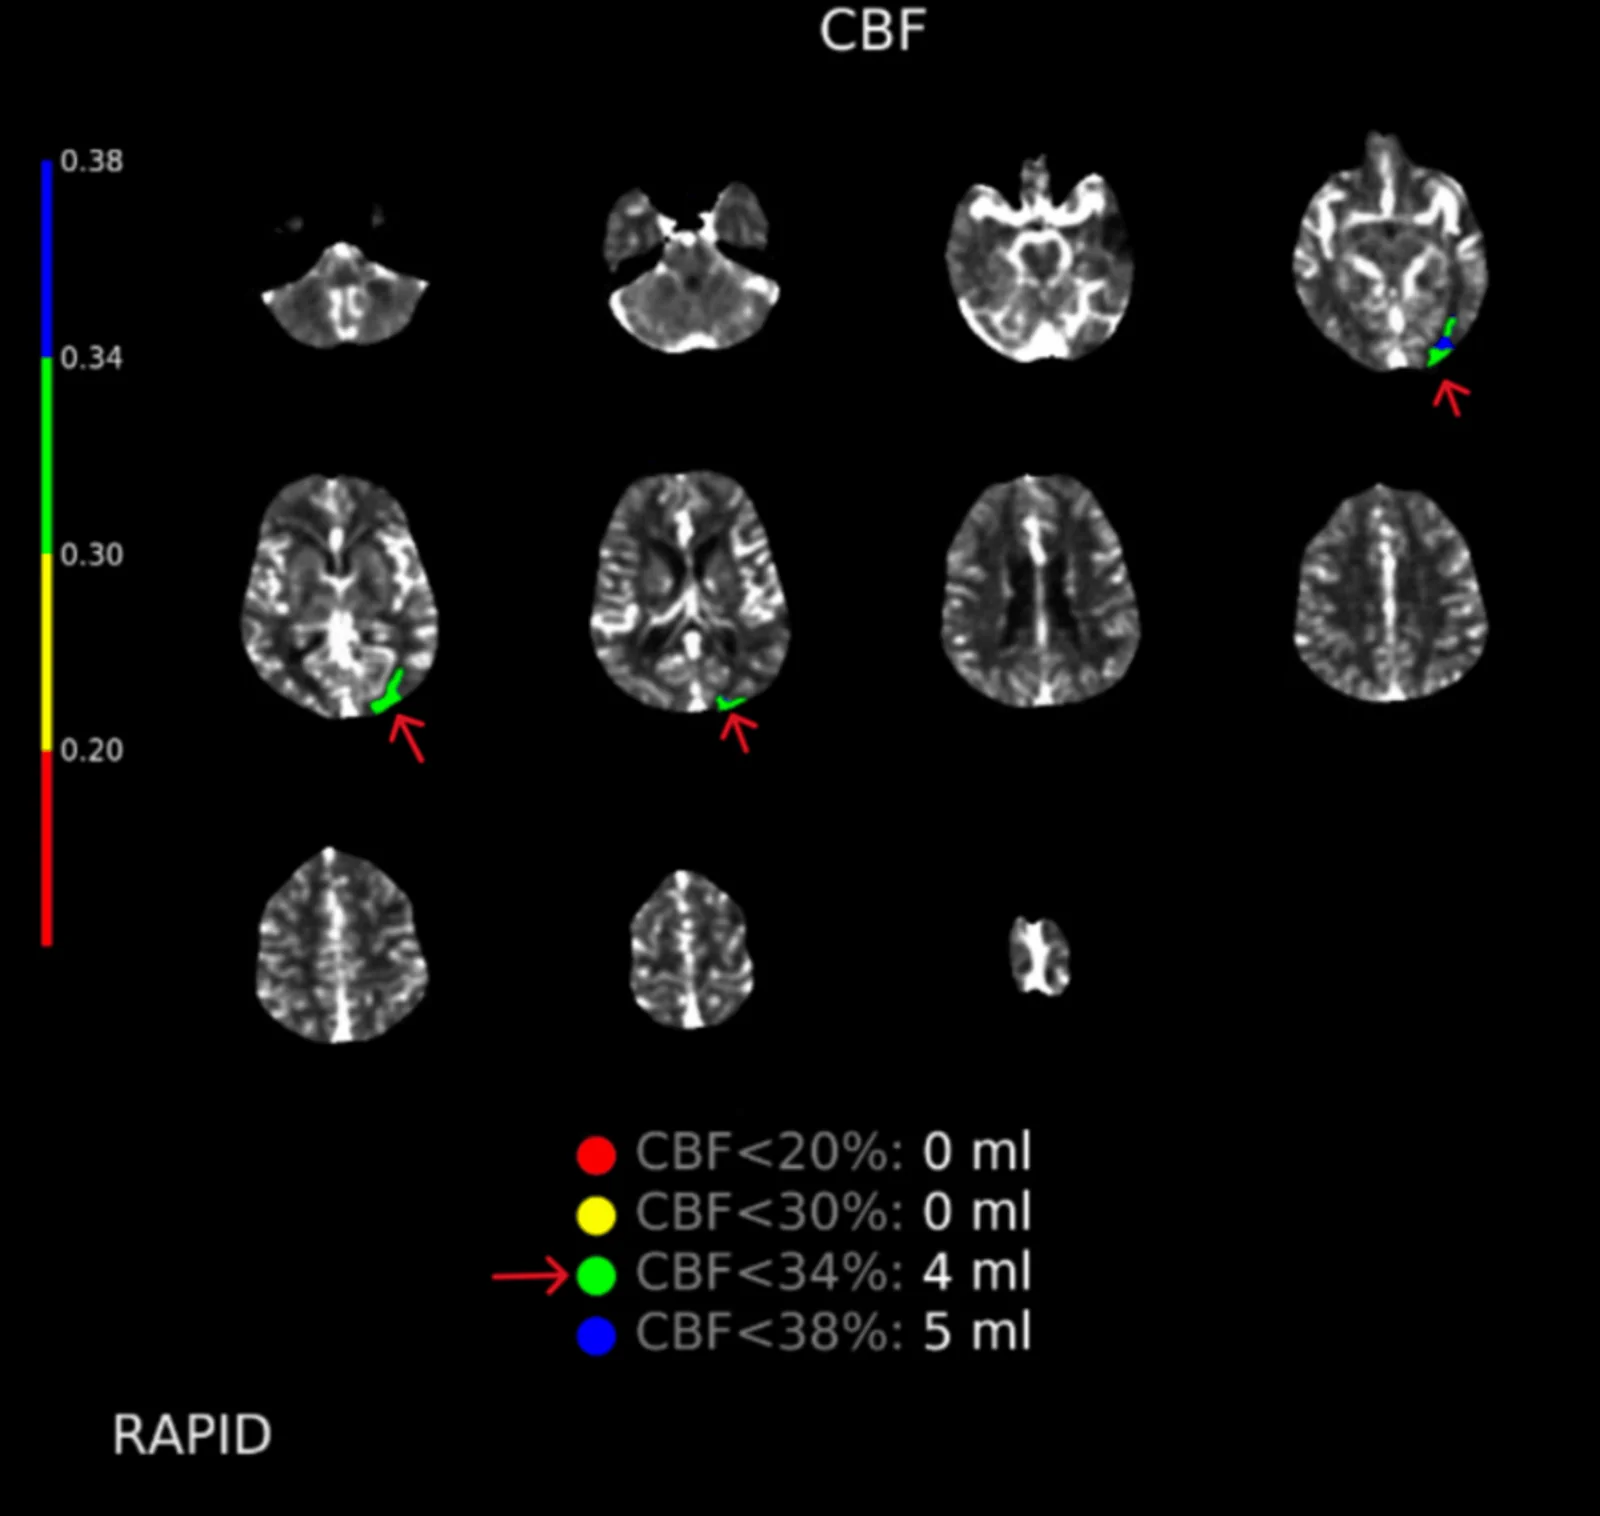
CT perfusion scans demonstrating a small region of reduced cerebral blood flow (CBF <34%), corresponding to an estimated ischemic core volume of 4 mL (as indicated by the red arrows).
